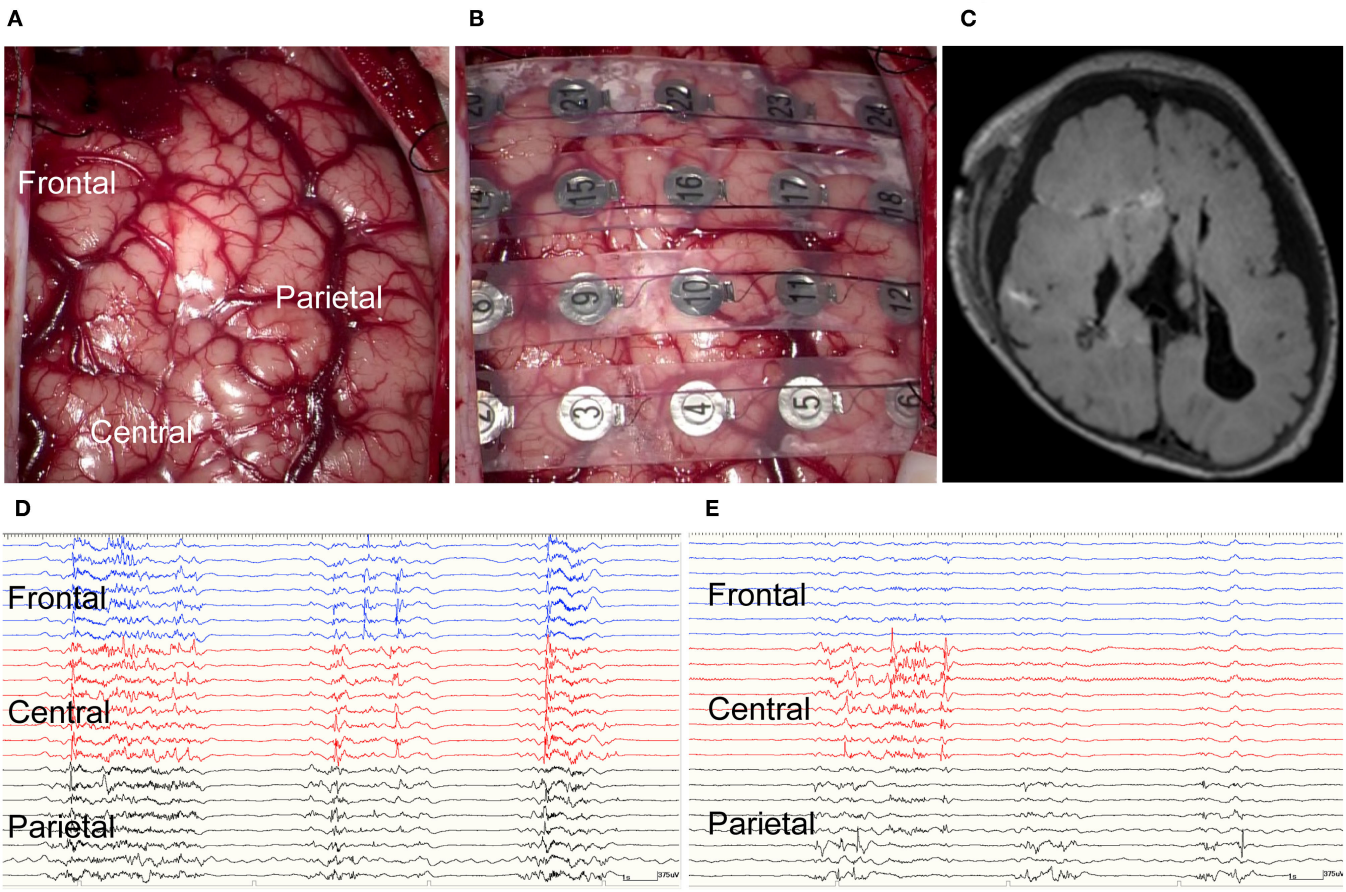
Intraoperative electrocorticography and postoperative imaging. (A) Intraoperative findings included absent Roland vein and ambiguous central sulcus. The sclerotic cortex was identified in the central area. (B) Intraoperative electrocorticography. (C) Postoperative axial fluid-attenuated inversion recovery magnetic resonance imaging (FLAIR MRI) demonstrated the disconnection line. (D) Intraoperative electrocorticography before subtotal hemispherotomy. (E) Intraoperative electrocorticography after subtotal hemispherotomy.
